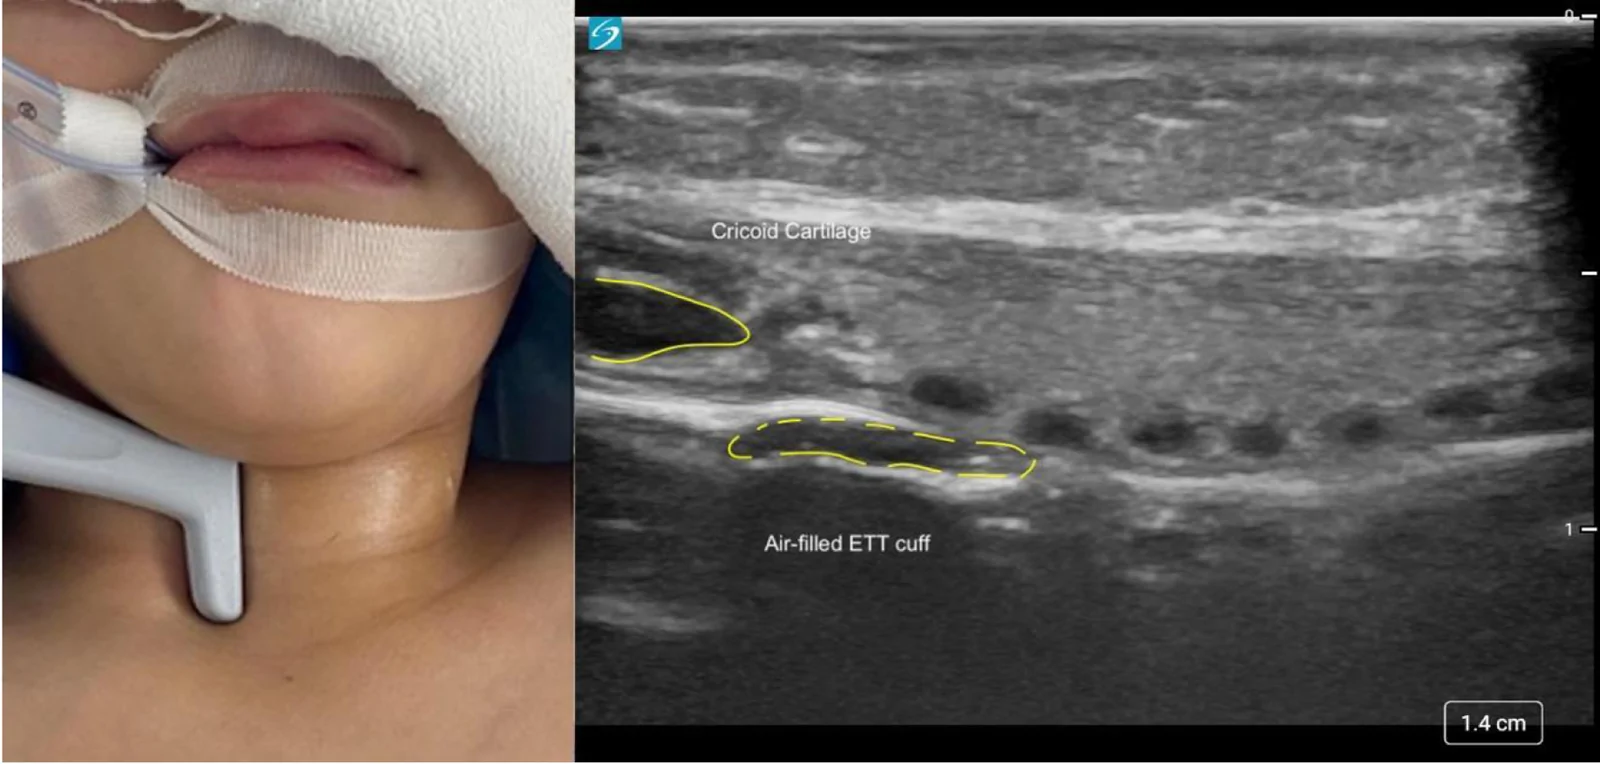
High-frequency linear probe placed on the anterior neck in the para-sagittal, long-axis plane (left) for airway ultrasonography. The cricoid cartilage and tracheal rings appear as anechoic structures. The anterior row of tracheal cartilages (anechoic structures) in the longitudinal plane is sometimes referred to as a “string of beads.” The air-filled cuff of the endotracheal tube also appears as an anechoic structure deep to the tracheal cartilages in a two-dimensional plane.
